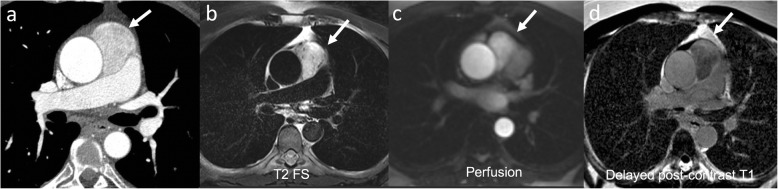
A 57-year-old male with chest pain. Axial (a) CTA image shows hypervascular mass between the ascending aorta and the main pulmonary artery (arrow). Further characterization with MRI (b–d) was performed, the mass had bright T2 signal with increased perfusion and wash out on delayed images. Pathology confirmed paraganglioma
